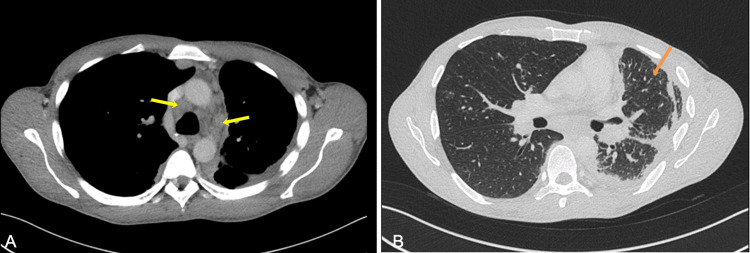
Follow-up chest CT scan after three months of anti-tuberculosis treatment, transverse plane. Image A shows mediastinal lymphadenopathies (4R and 4L) that remained stable despite the treatment (yellow arrows). Image B reveals a new nodular pattern in the left lung with perilymphatic distribution (orange arrow).
